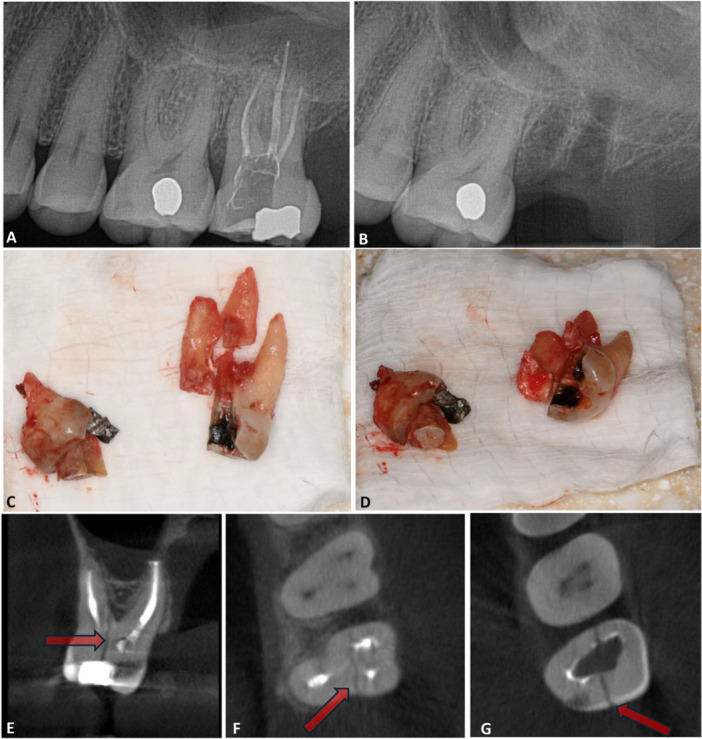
A case of a vertical root fracture in a patient's upper left second molar. Picture A: Pre‐operative periapical radiograph. Picture B: Post‐operative radiograph after extraction. Picture C: Fractured tooth in a similar orientation to the periapical radiograph. Picture D: Occluso‐apical view of the split tooth segments. Picture E: Sagittal view of the CBCT scan, the arrow indicates a VRF from the occlusal surface to the bifurcation area. Picture F: Axial view of the same CBCT at the level of the bifurcation area, the arrow indicates the area of the VRF where it terminates. Picture G: Axial view of the same CBCT at the level of the pulp chamber area, the arrow indicates the area of the VRF.
